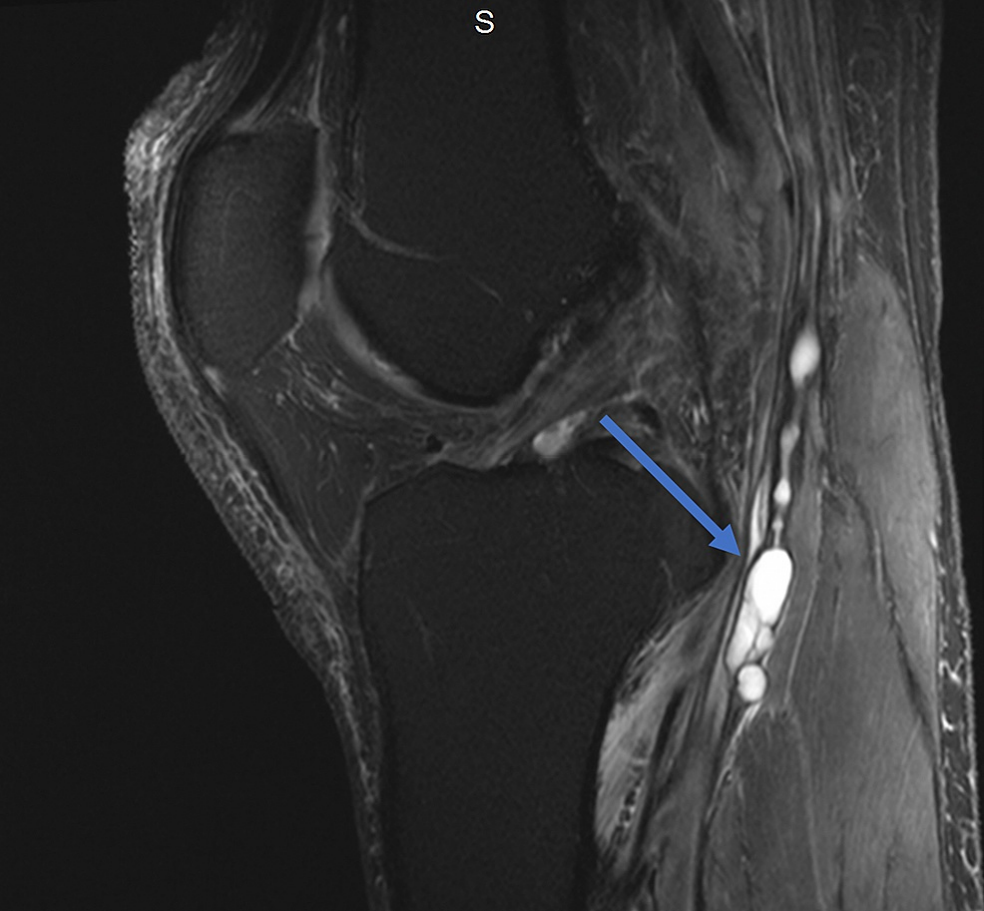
Sagittal T2-weighted TSE fat-saturated image (transverse view) demonstrating signal hyperintensity due to multilobulated tubular cysts within the tibial nerve TSE, turbo spin echo
